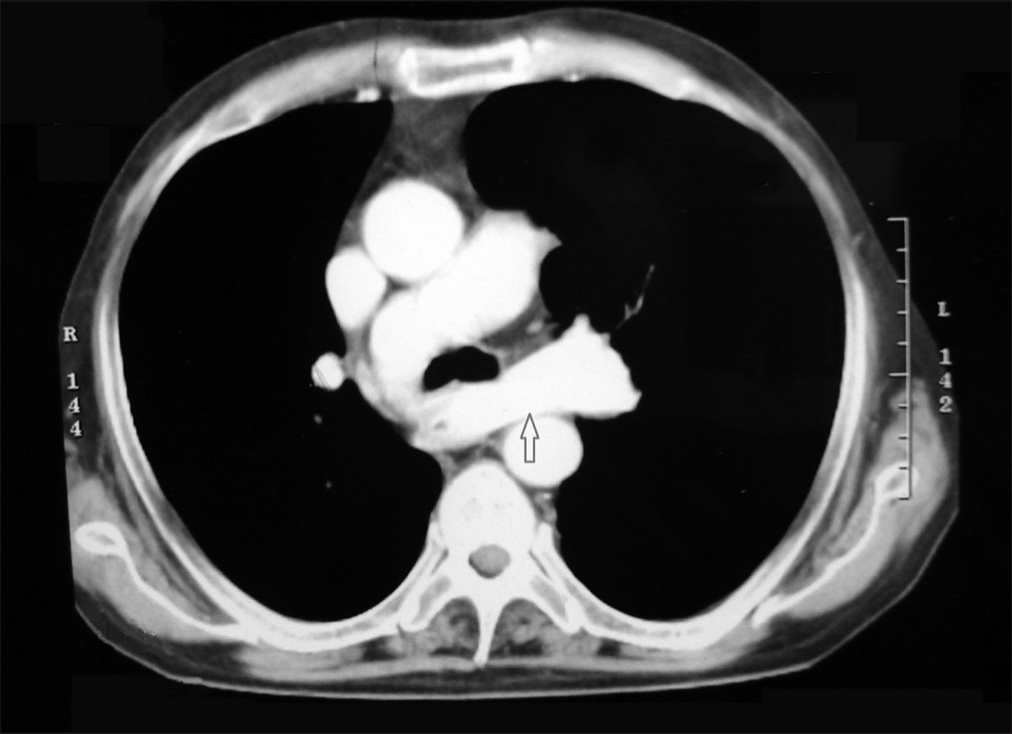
Section of CT thorax showing aberrant course of left pulmonary artery (arrow)
